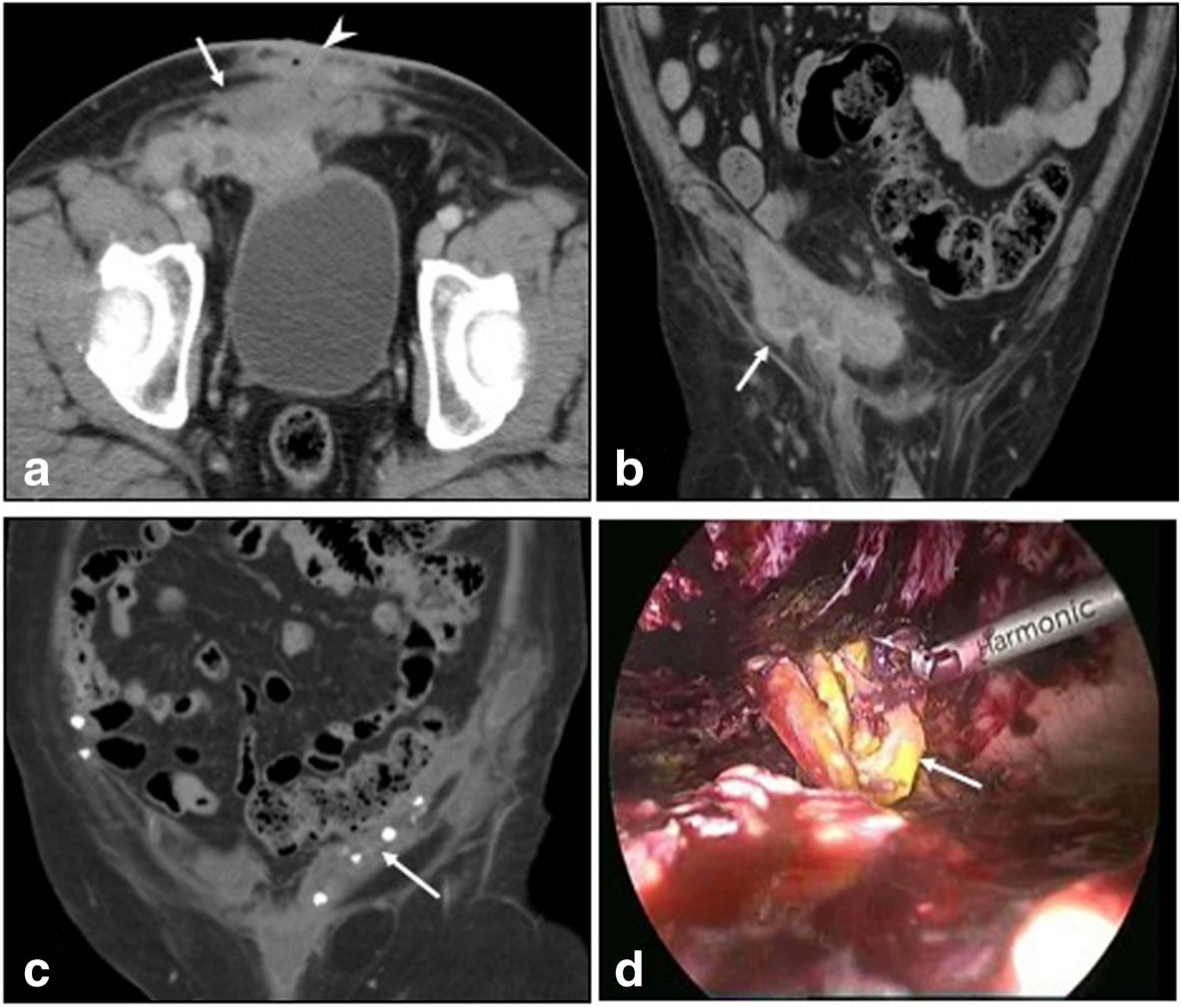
a, b CECT in axial and coronal sections show infected right inguinal hernia mesh repair with collections (arrows) and sinus tracks (arrowhead). Culture yielded *Mycobacterium chelonae*. c Coronal plain CT in another patient shows soft tissue thickening and collection along the mesh tackers (arrow). d Laparoscopic retrieval of infected mesh (arrow) which was covered with pus and granulation tissue
